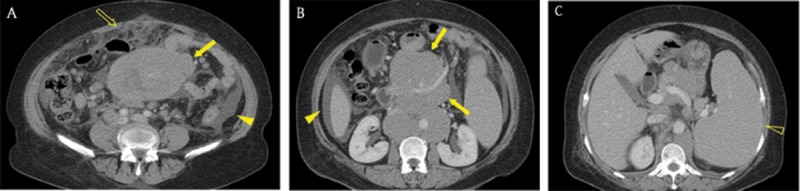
A 60-year-old woman with mantle cell lymphoma. (A, B) Axial portal venous phase CT images revealed omental haziness (open arrow) and ascites (arrowhead). Confluent and bulky lymphadenopathy (arrows) was located on both sides of the mesenteric vessels, representing the ‘sandwich sign’. (C) The size of the spleen (open arrowhead) was increased.
